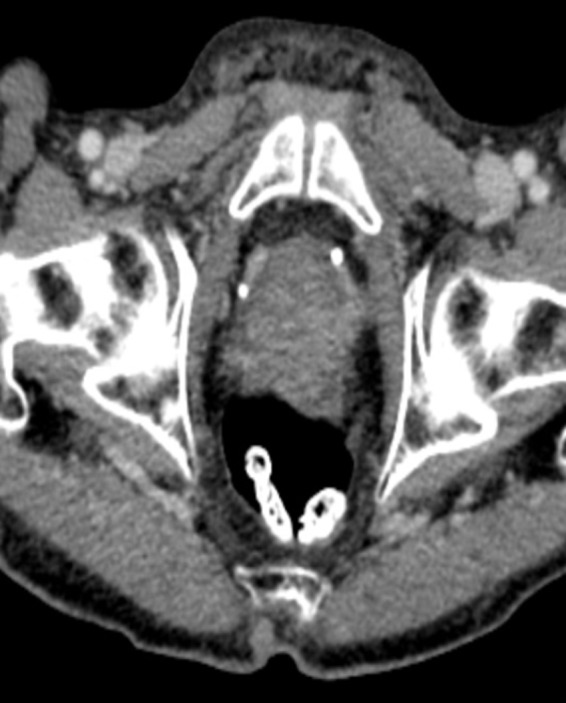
Axial postcontrast CT shows mild‐to‐moderate enlargement of the prostate gland.
